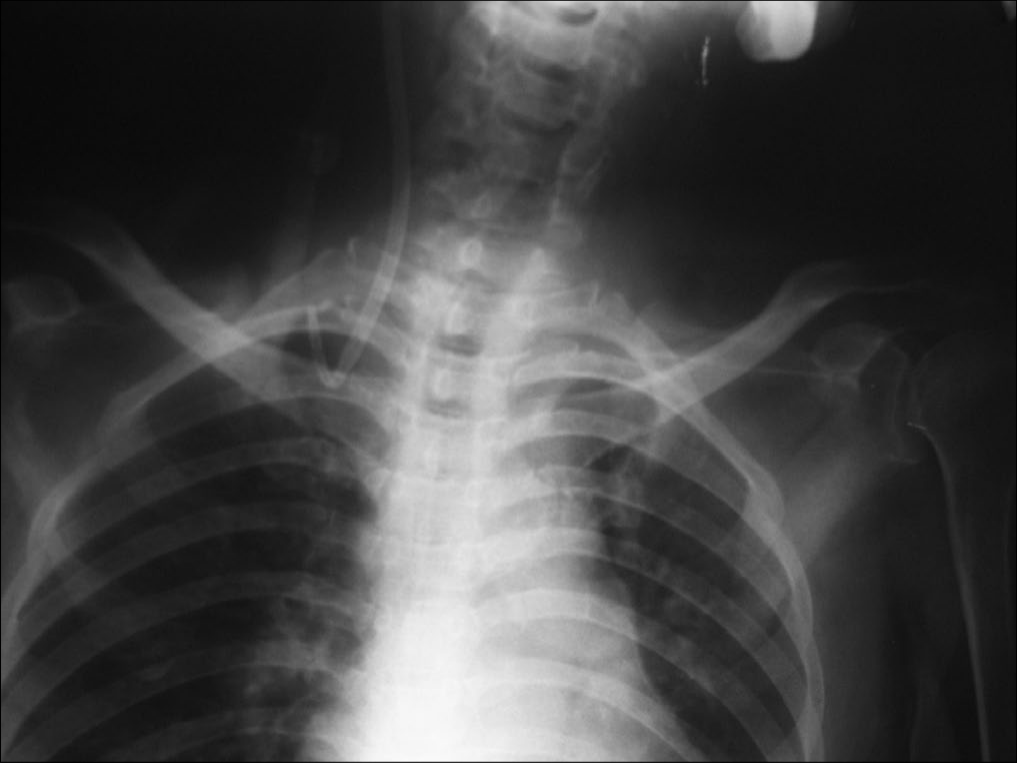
Chest X-ray showing the misplacement of right internal jugular hemodialysis catheter
